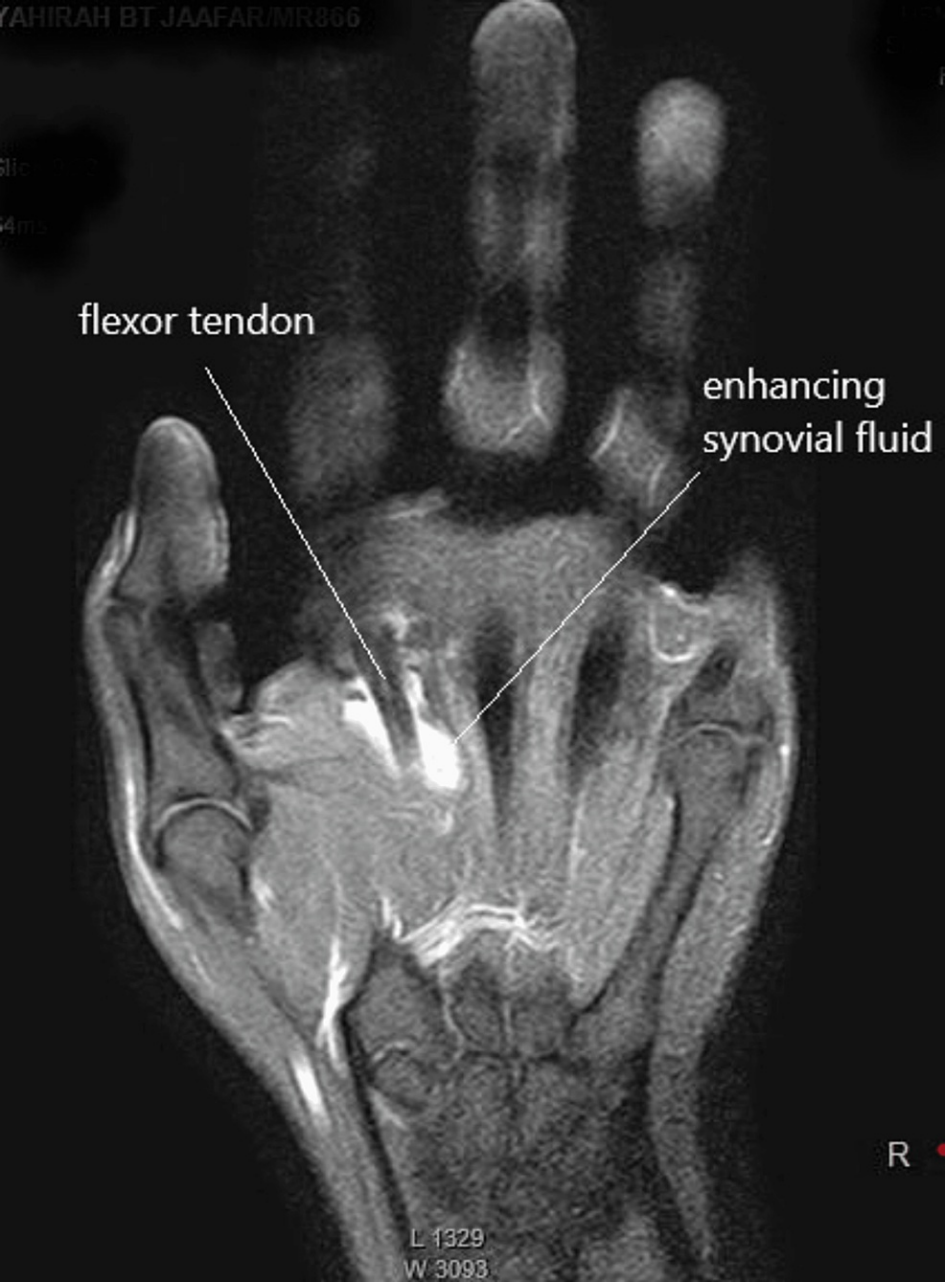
MRI coronal view of the left hand. MRI of the left index finger showed a hyper-intensified signal around the flexor tendon. MRI: magnetic resonance imaging
